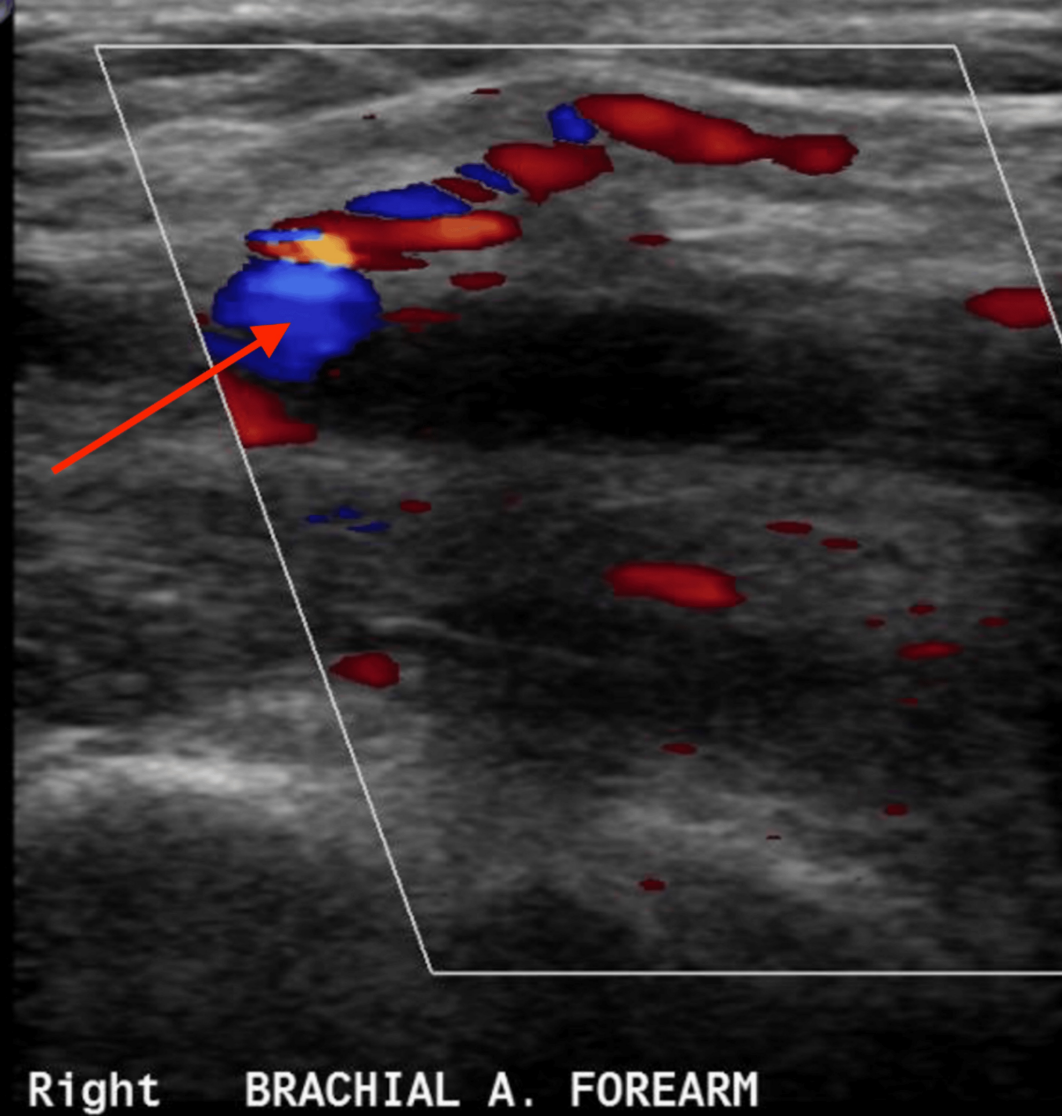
Color Doppler ultrasound Color Doppler ultrasound of the right brachial artery revealed a thrombus, seen as a round anechoic structure exhibiting bidirectional flow on Doppler imaging, producing the classic “yin-yang” sign. The lesion was located adjacent to the brachial artery in the forearm, consistent with turbulent flow within a contained arterial wall defect. These findings are suggestive of a brachial artery thrombus, likely secondary to prior trauma or vascular intervention.
